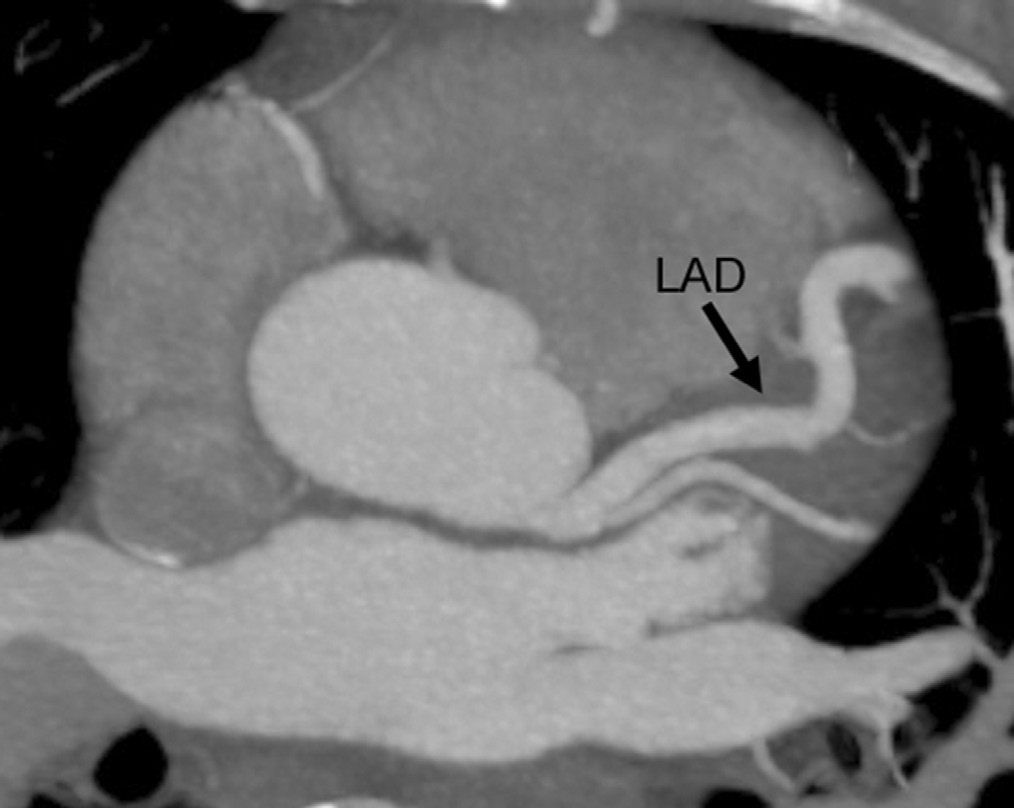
CT coronary angiogram superior axial maximum intensity projection Figure showing a large and tortuous left anterior descending artery (LAD) originating from the left coronary cusp and situated in its usual anatomical location.
